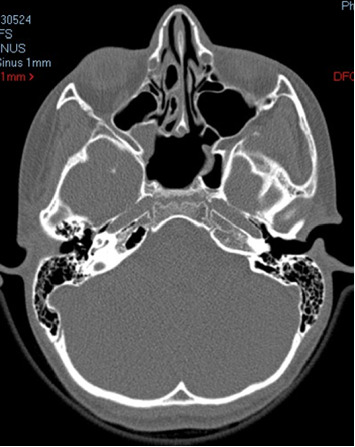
Brain CT in axial view with complete aeration of the sphenoid sinus.
